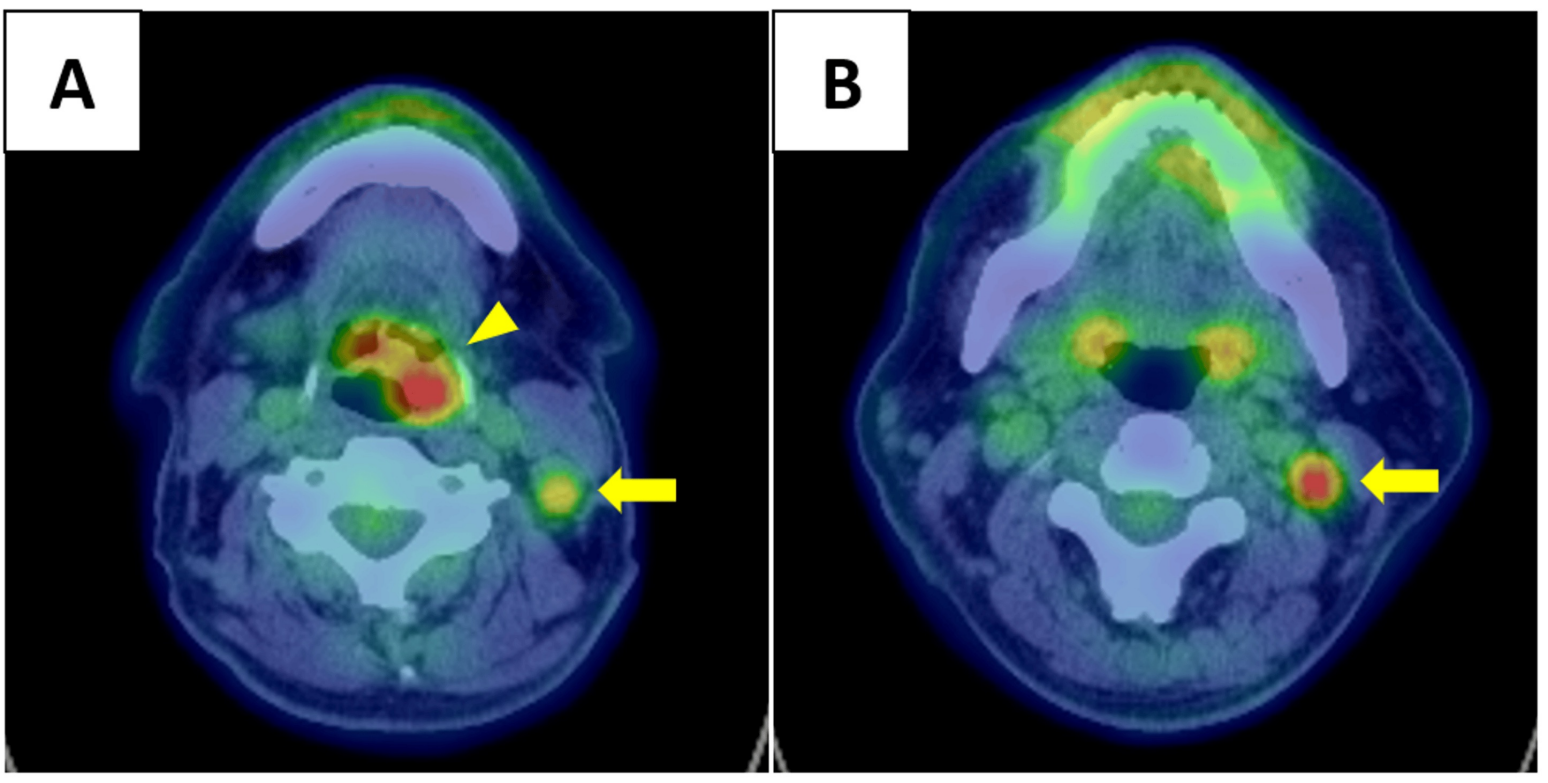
Axial view of positron emission tomography-computed tomography (PET-CT) Increased fluorodeoxyglucose (FDG) uptake is observed in the lesion on the left side of the epiglottis (yellow arrowhead) and in multiple lymph nodes in the left cervical region (yellow arrows), with a maximum standardized uptake value (SUVmax) of 8.1. No distant metastasis is identified.
